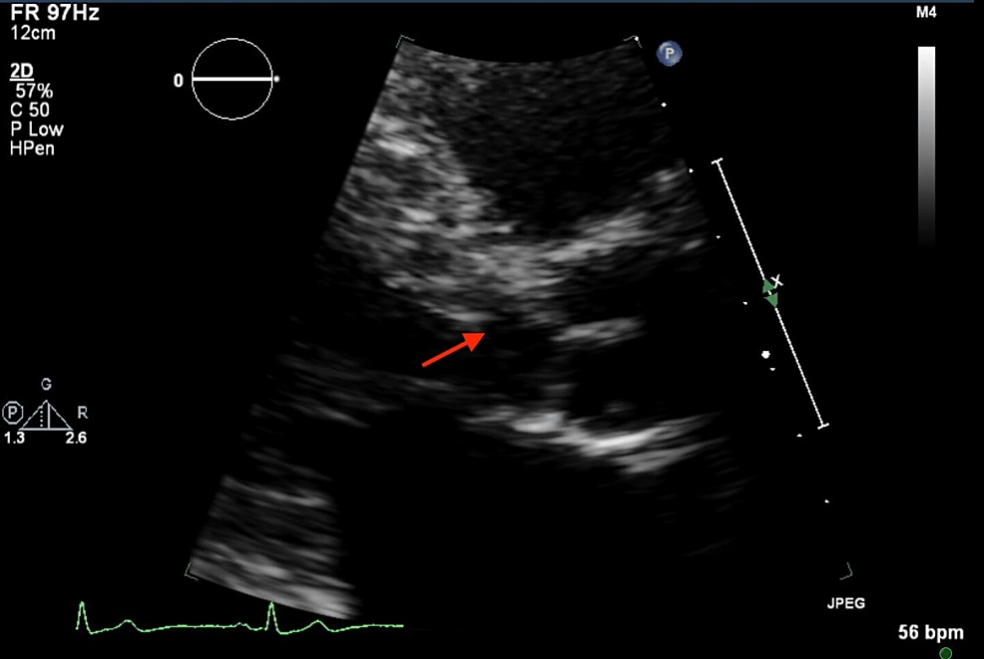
Transthoracic echocardiogram (parasternal long axis view, zoomed in) showing subaortic membrane (red arrow).
